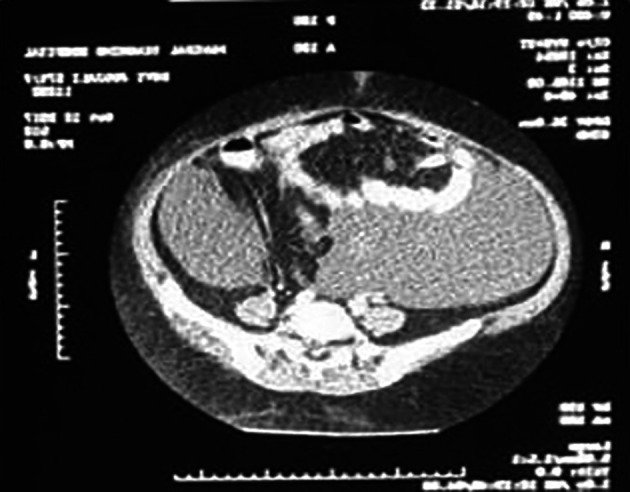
CT scan of the abdomen and pelvis showing the complex ovarian cyst.
